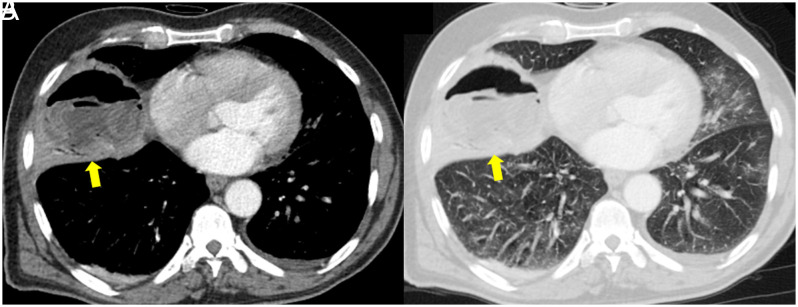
Axial section of chest CT in a 68-year-old male patient showing a complicated hydatid cyst in the right middle lobe of the lung as seen in the mediastinal (A) and lung (B) windows (arrow).
